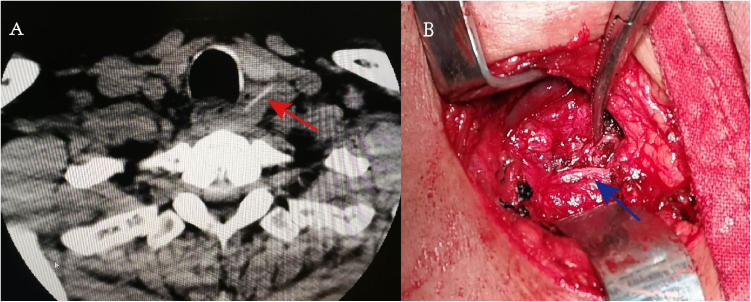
The CT scan showing a linear object of high density in the left tracheoesophageal groove (A), the left RLN was kept intact during the fishbone removal surgery (B).
